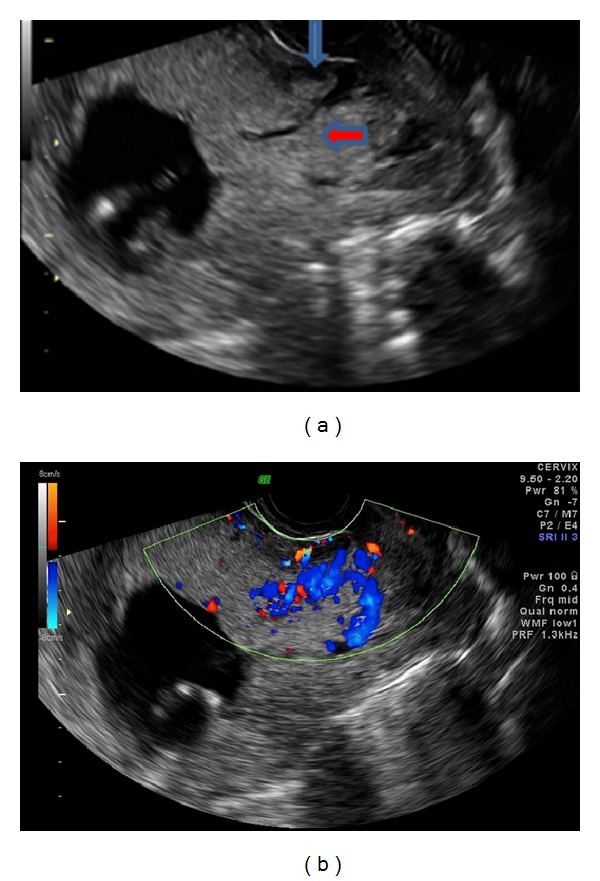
(a) Transvaginal scan at 13 weeks' gestation demonstrating placental lacunae (red arrow) and loss of placental-myometrial interface (blue arrow). (b) Colour Doppler demonstration of vascular placental lacunae with turbulent flow.
